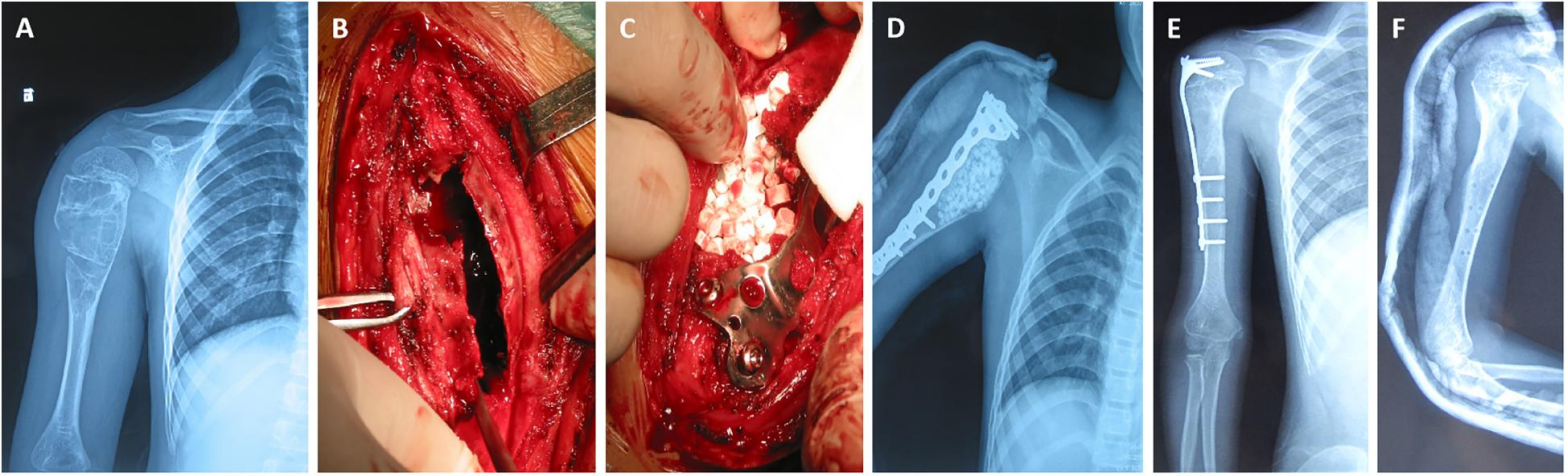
Curettage and bone grafting combined with electrocautery and drill, supplemented with plate fixation for the treatment of pediatric humeral aneurysmal bone cysts. (A) preoperative x-ray showed eccentric osteolytic changes; (B) curettage by electrocautery and drill, and (C) bone grafting; (D,E) x-ray at 1 week and 1 year postoperatively showed no complications such as hardware failure or bone resorption; (F) the plate fixation was removed at 2 years postoperatively.
